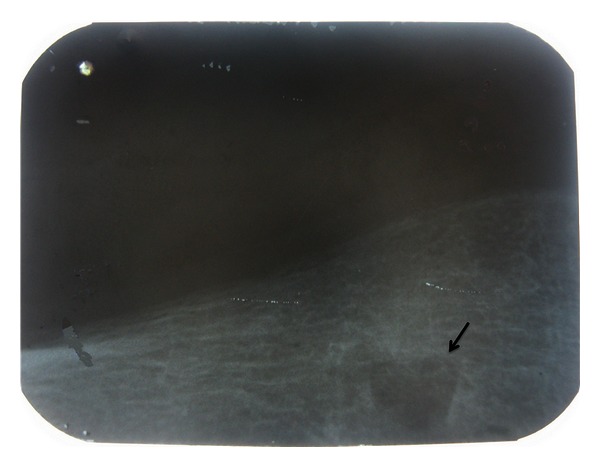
Postsurgical intraoral periapical radiograph showing the enlarged mental foramen with complete absence of the foreign material.
